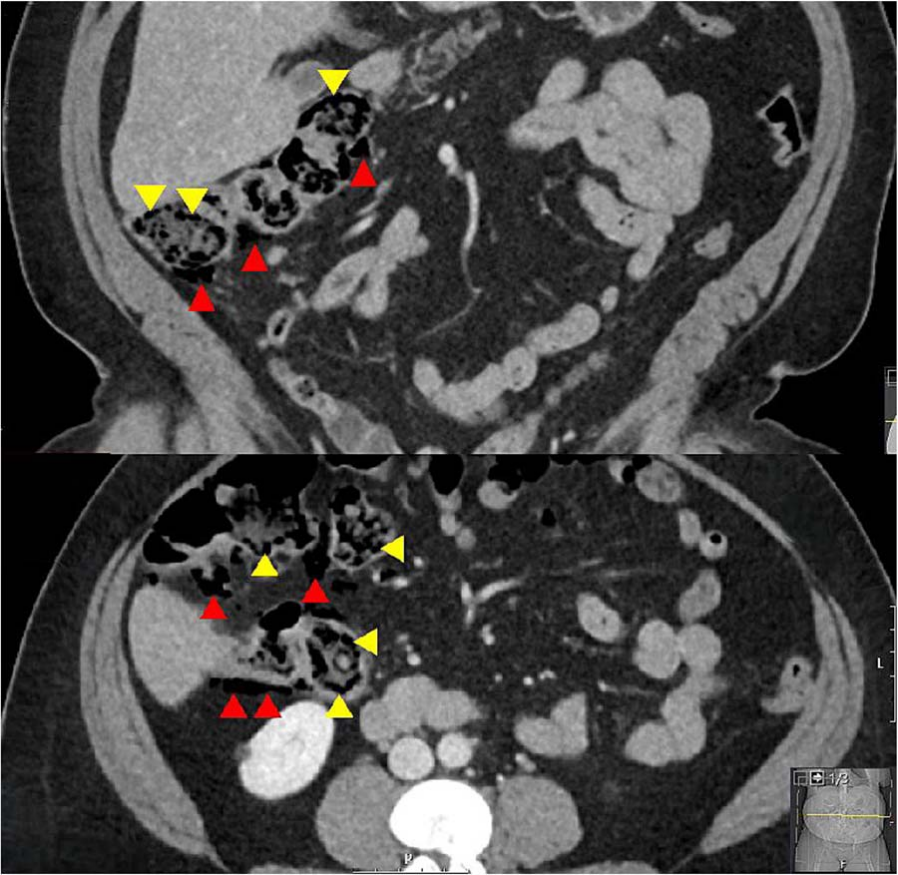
Coronal and axial CT slices showing pneumatosis in the right colon. Arrowheads demonstrate subserosal and submucosal gas.
